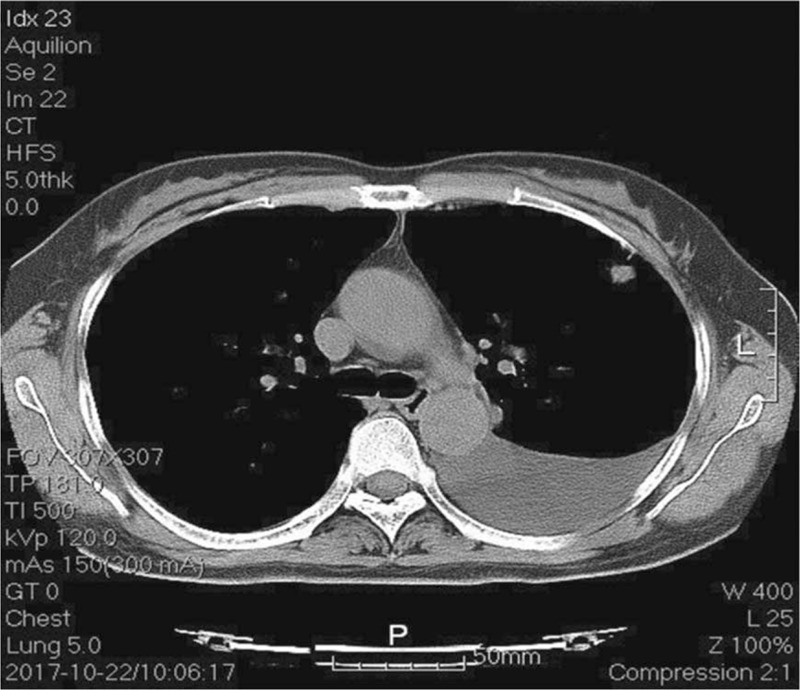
Computed tomography (CT): (1 axial CT images) results showed the presence of nodules in the left upper lobe and a small amount of pleural effusion on the left side in the lung.
